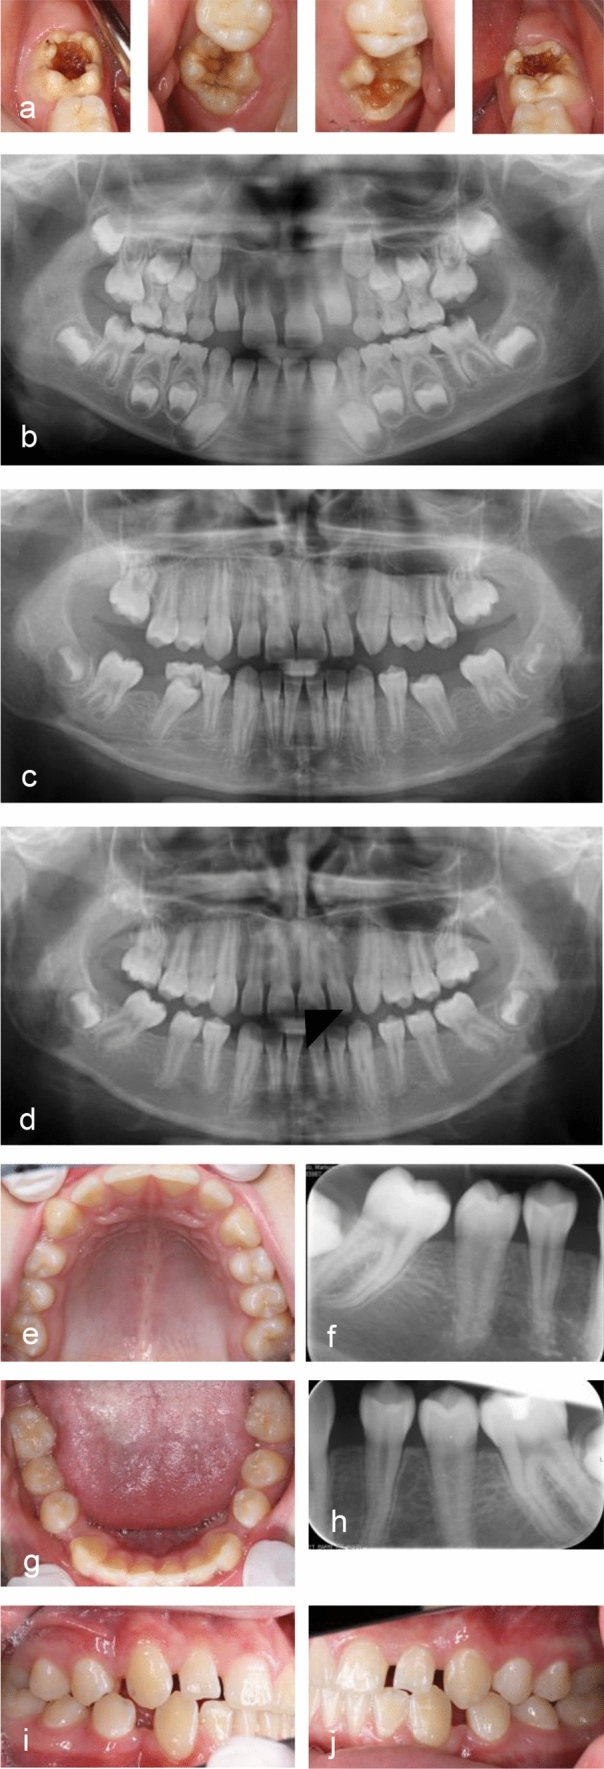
Picture series of child having all FPM extracted at 9.5 years age. a Clinical photographs before treatment show that all FPM have severe decay. The child experienced pain from the molars. b Panoramic x-ray at 6 years. Ectopically erupting upper FPM. Posteruptive breakdown and caries. The orthodontist did not recommend extraction and the teeth were treated with semipermanent fillings until extraction. c Panoramic x-ray at 11 years. d Panoramic x-ray at 13 years. Full space closure is seen in the maxilla. In the mandibula, there are spaces on both sides and tipping of tooth 47. e–j At age 15, the child has no subjective treatment need and is happy with his teeth. Spaces are seen on both sides. The tipping of 47 is somewhat reduced since the previous control and 48 is present. e, g: Clinical photographs, occlusal views. f, h Apical radiograph from 4th and 3rd quadrant. i, j Clinical photographs buccal view both sides
